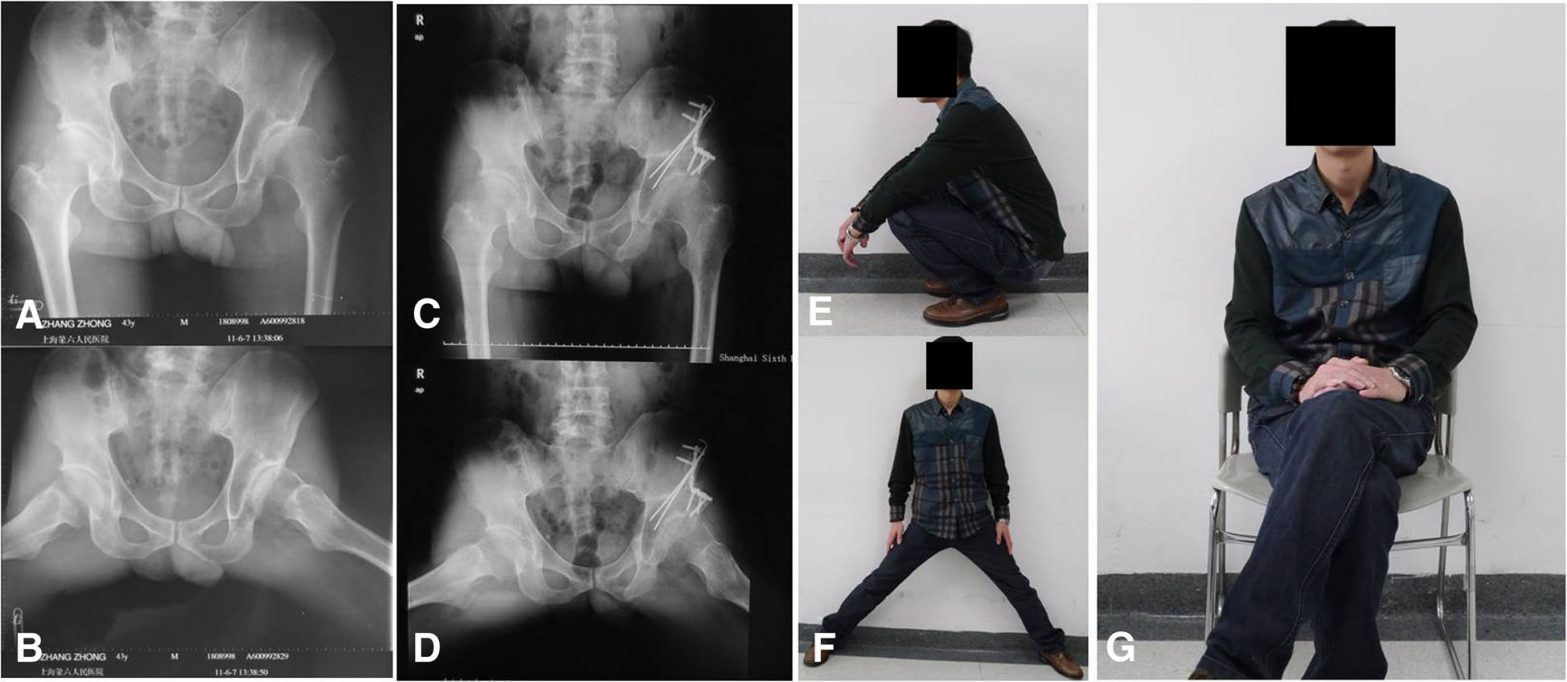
Radiographs of a 41-year-old male patient admitted for gradual pain in the left hip and limping for 8 months who was diagnosed with developmental dysplasia of the left hip (a) and Tönnis grade 1 osteoarthritis on radiography (b). Radiographs after modified triple pelvic osteotomy for the left hip was performed with the patient under general anesthesia (c) and at 6 months of follow-up (d). It was observed that the coverage rate of the femoral head significantly increased, the operative area healed well, pain and limping disappeared, and the range of hip motion improved. The range of hip motion of the same patient at 6 months after the surgery (e, f, g)
